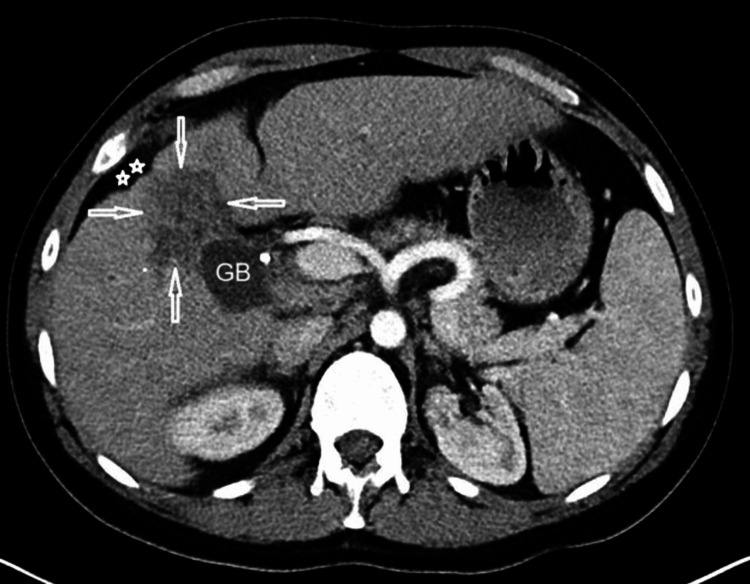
Selected contrast-enhanced computed tomography (CECT) image of the liver and gallbladder (GB) demonstrated the lesion (arrows) on segment IV as heterogeneous area with liver capsular retraction (stars)
